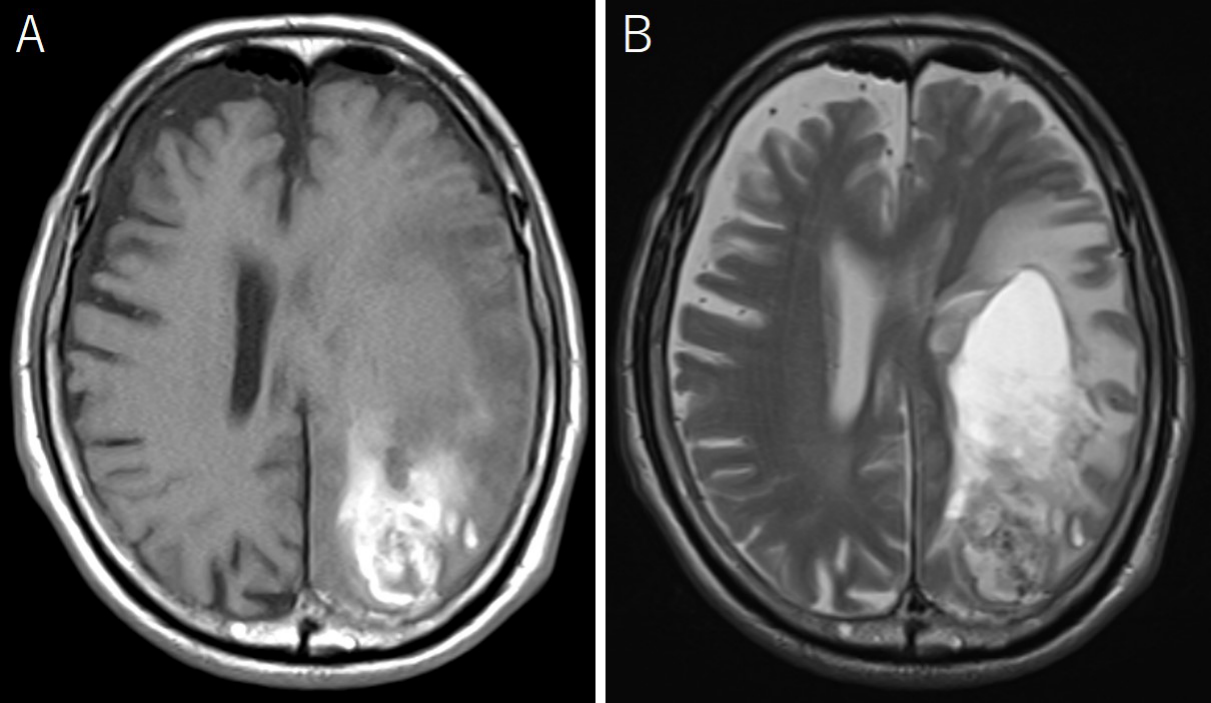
Magnetic resonance images on postoperative day 12; cyst showed iso intensity in the T1-weighted image (A) and marked high intensity in the T2-weighted image (B).
